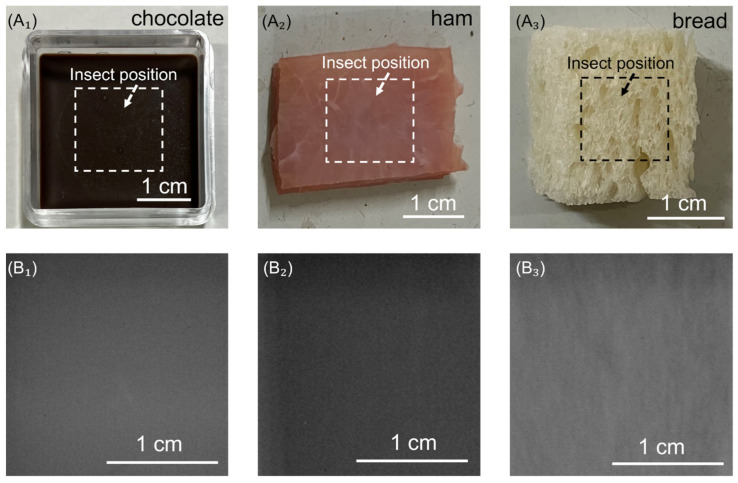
Sample contaminated with insect (A_1_–A_3_). Insect detection results using X-ray transmission system (B_1_–B_3_).
